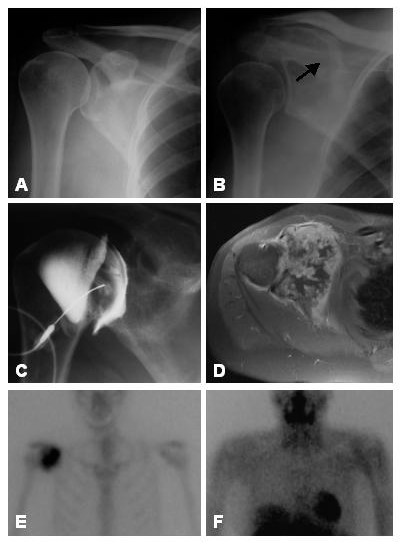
A Initial plain radiographs of the shoulder were unremarkable. B Repeat radiographs after two years of failed treatment, showing an irregular mixed lytic and sclerotic lesion destroying the coracoid process of the scapula (arrow), which was not appreciated. C Arthrogram performed prior to hydrodilatation similarly showing the destructive process, which remained unnoticed. D Axial T1-weighted post-contrast MR image showing a heterogenous contrast-enhancing lesion destroying the glenoid and extending into the gleno-humeral joint. The lesion is lobulated and loculated with central areas of lower signal intensity, suggestive of a chondroid lesion. E Bone scan showing increased uptake in the area of the lesion on the delayed static image. F Thallium functional scanning showing no retention of thallium by the lesion at 4 hrs. Biopsy confirmed low-grade chondrosarcoma.
